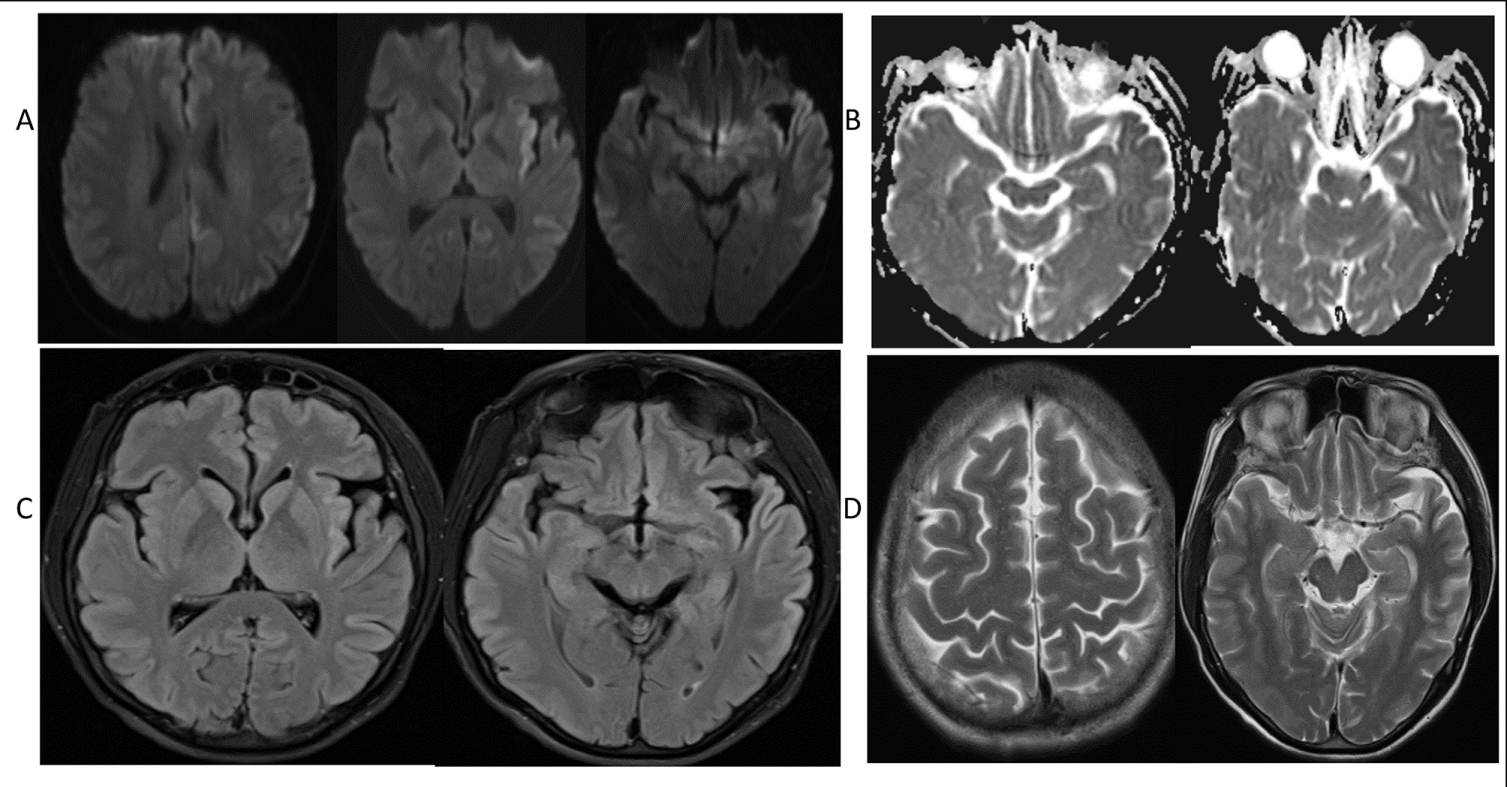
Diffusion Weighted Images (DWI); diffuse cortical injury evident by diffusion restriction also involving the corpus striatum(1 A); Susceptibility Weighted Images (SWI); diffuse cortical injury evident by diffusion restriction(1B); FLAIR; Diffuse cortical hyperintensity(1 C); T2 WI, evidence of mild volume loss in both cerebral hemispheres(1D)
